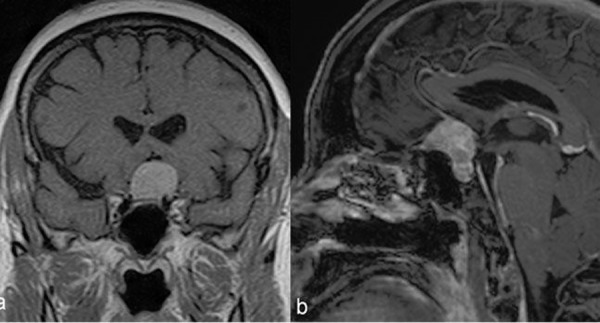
Picture showing a preoperative MRI scan showing the case of a tuberculum sellae meningioma.
